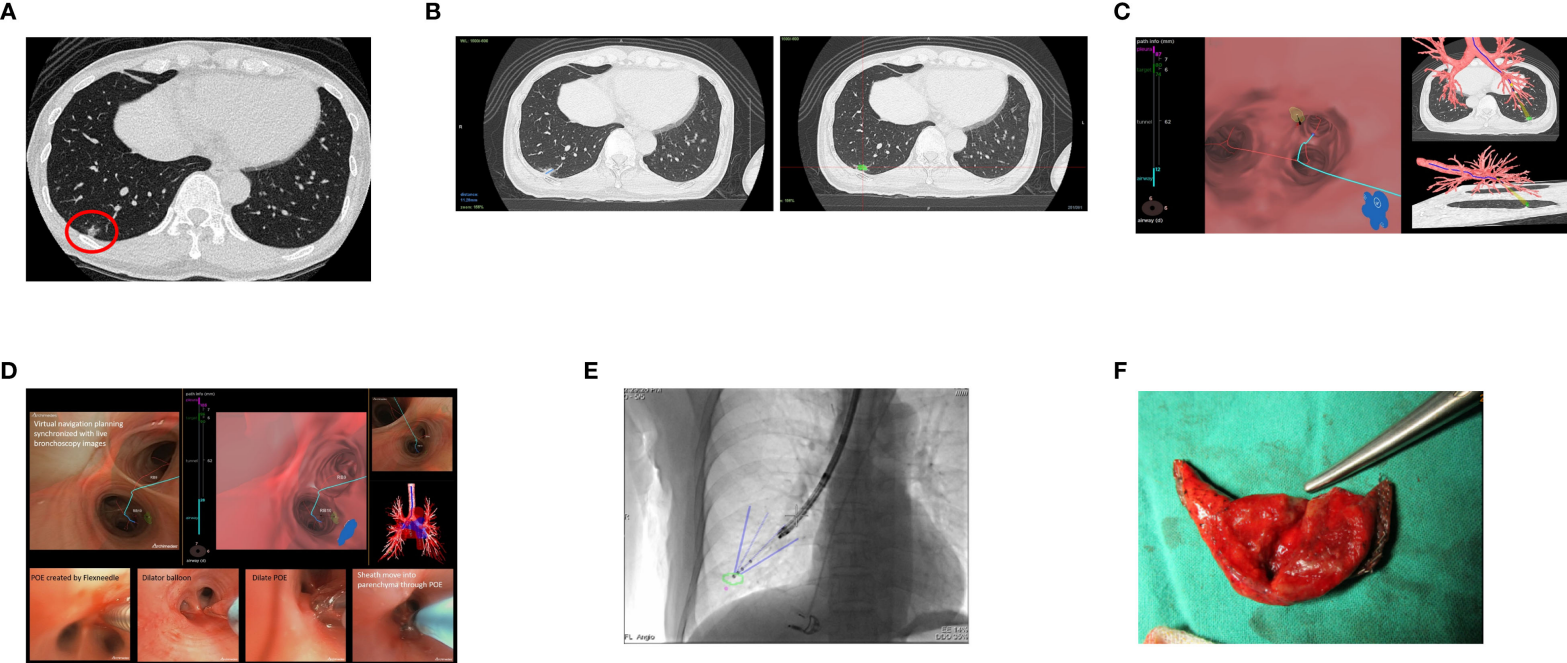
(A) Routine low-dose CT screening revealed a 1.1 mm part-solid pulmonary nodule in the right lower lobe (RLL) (red circle). (B) The Archimedes™ system localized the nodule in the right lower lobe (RLL), specifically in segment RB10. (C) The Archimedes™ system calculated a tunnel length of 61.5 mm and a distance of 7.3 mm from the pleura to the target. (D) Images during navigation to reach the target: Virtual navigation planning synchronized with live bronchoscopy images, creation of the point of entry (POE) using the FlexNeedle, dilation of the POE with a balloon dilator, and advancement of the sheath into the parenchyma through the POE. (E) CBCT was utilized to confirm precise localization. (F) Photo of the resected wedge tissue.
